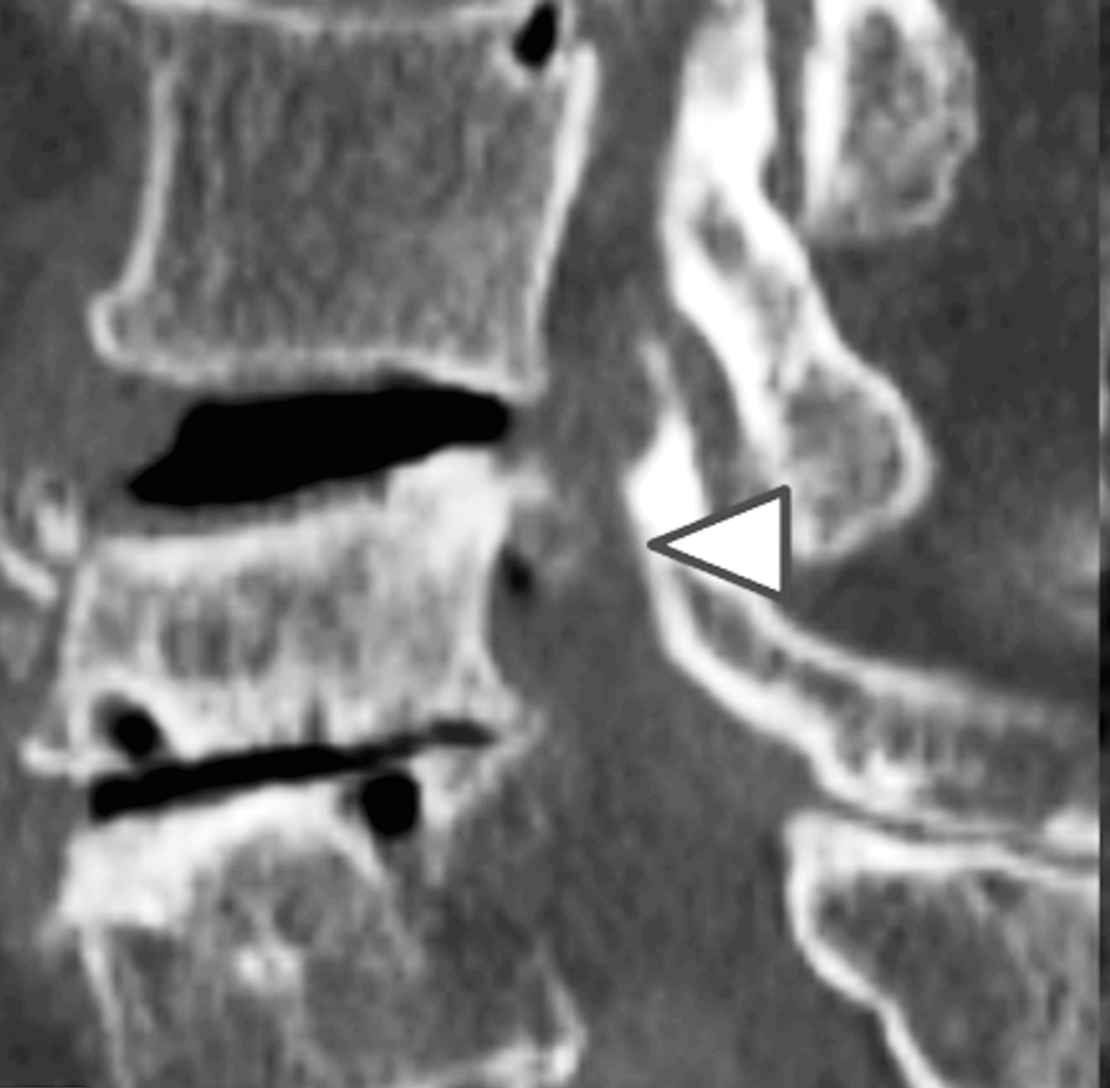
Sagittal view of lumbar 3D-CT Three-dimensional computed tomography (3D-CT) showed a retropulsed bone fragment (white arrowhead) with L3 OVF. OVF: osteoporotic vertebral fracture
